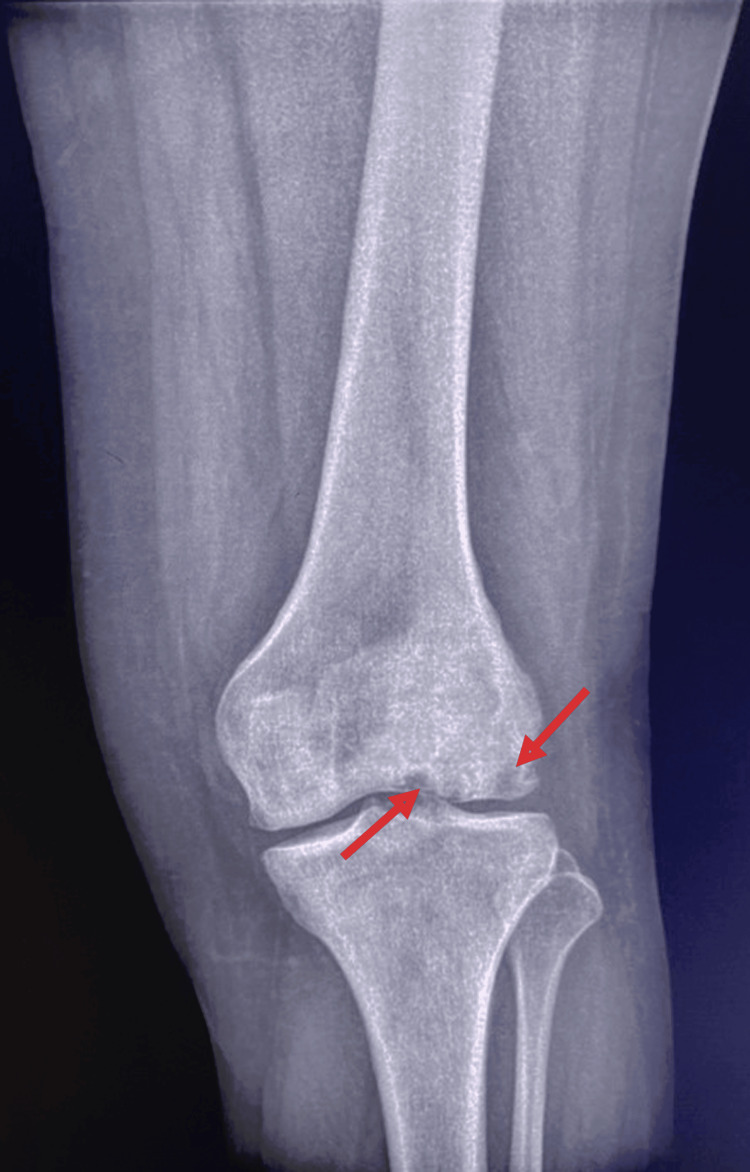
Radiograph of the left knee demonstrating osteolytic areas within the distal femur.
